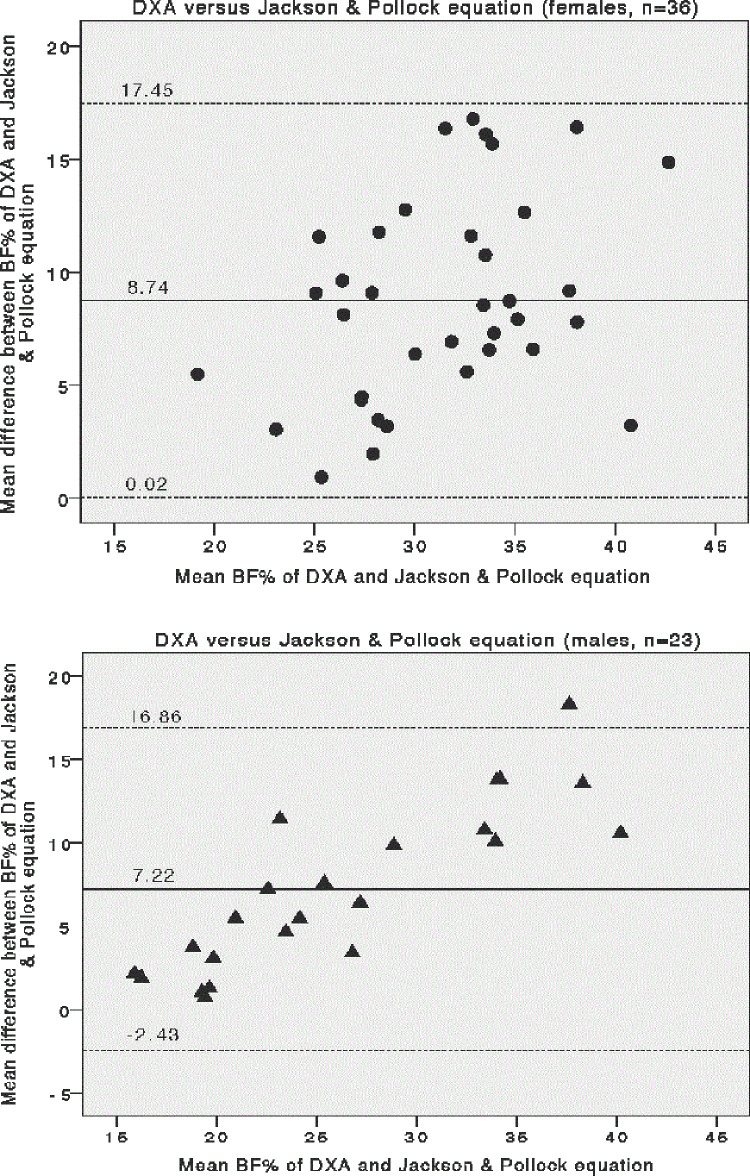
Comparison of BF% measured by DXA and Jackson & Pollock 7-site skinfold equation and displayed as Bland–Altman plots in females (a) (black circle) and males (b) (black triangle). The central line represents the mean bias between measurements. Dotted lines represent upper and lower 95% limits of agreement. Y-axis represents difference of BF% measured by DXA minus BF% measured from skinfolds. Abbreviations: BF%, body fat percentage; DXA, dual energy X-ray absorptiometry.
